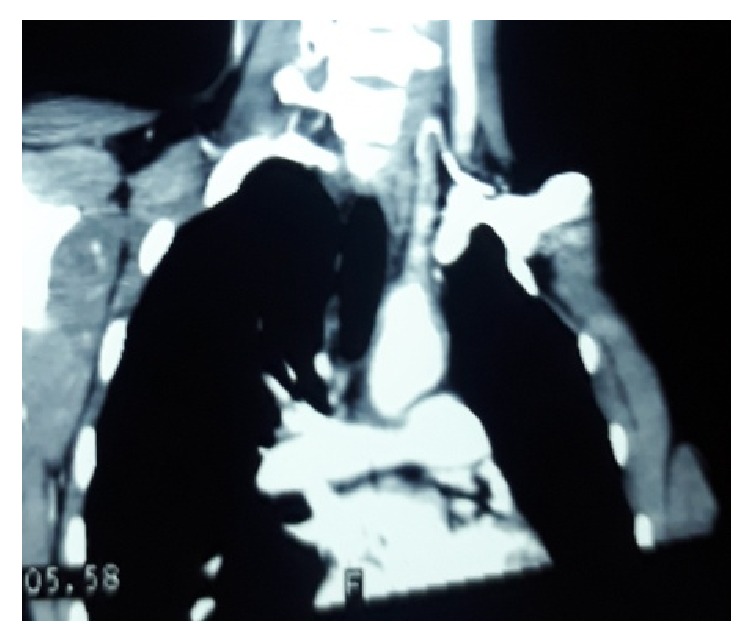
Computed tomographic aortogram revealed circumferential thickening of arch of aorta, brachiocephalic trunk, and right common carotid.
